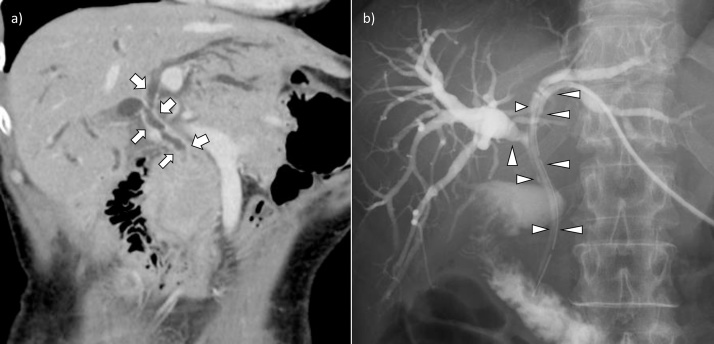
Enhanced CT showing extensive cholangiocarcinoma with wall thickening and biliary stenosis (arrow) (a). PTBD cholangiography showed irregularity at the same lesion (arrowhead) (b).
